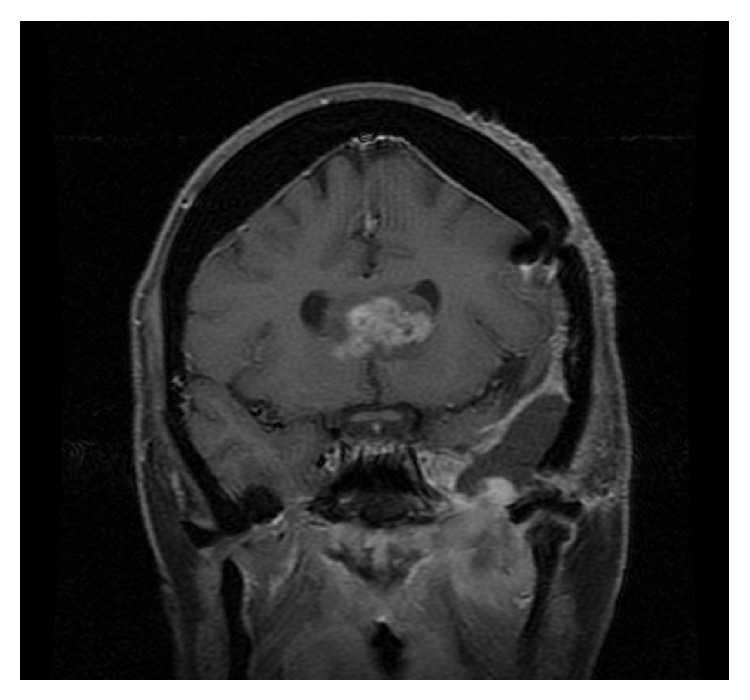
Postoperative (8-month) follow-up T1-WI axial MRI demonstrates recurrent local tumor, likely from anaplastic astrocytoma component, and distant spread to the anterior part of corpus callosum.
